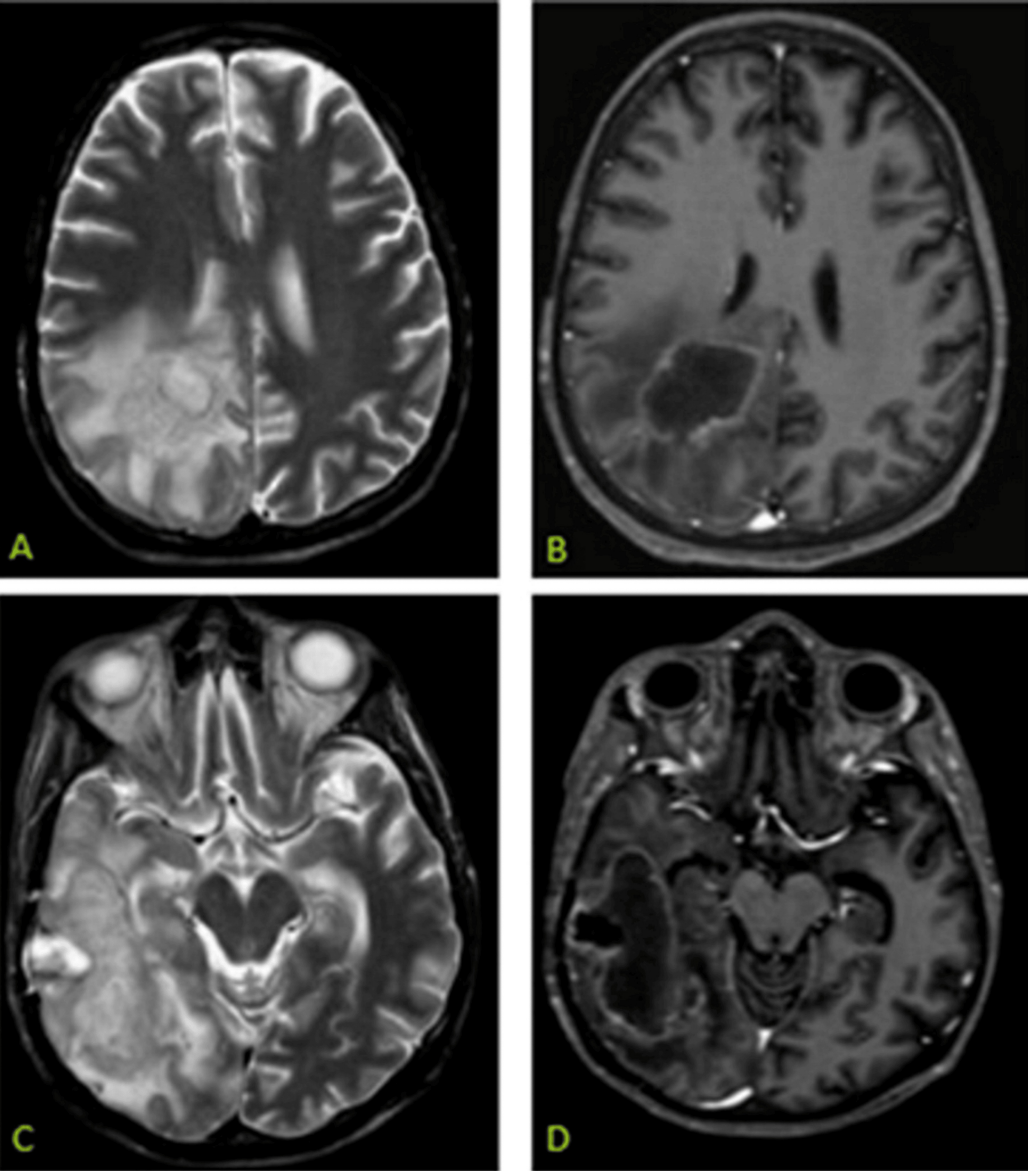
An MRI scan obtained after one month. T2 and post-contrast images show an increase in the size of the lesions and corresponding vasogenic edema.
